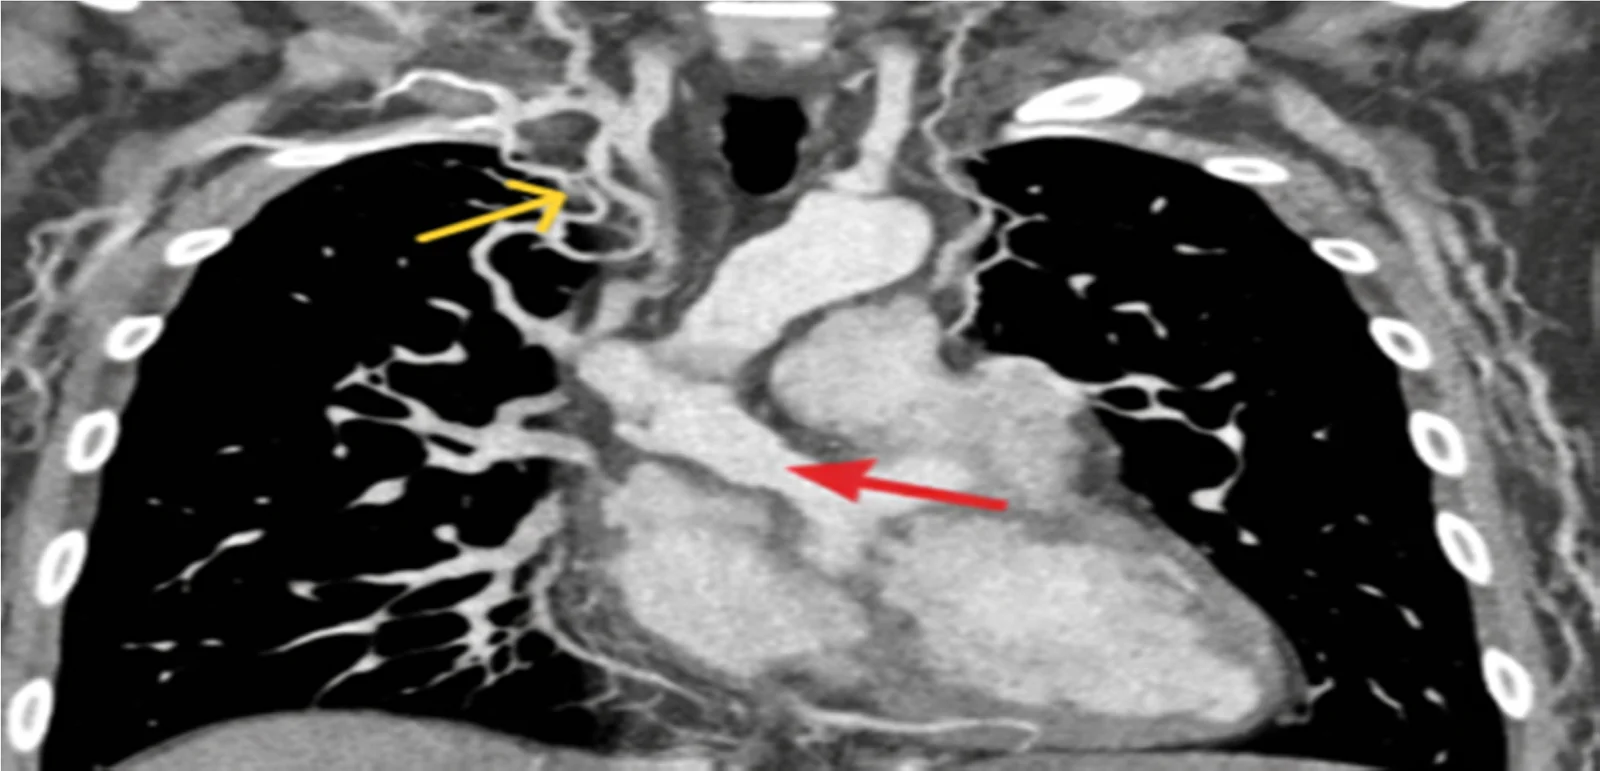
Coronal contrast-enhanced CT pulmonary angiography (CTPA) demonstrating a filling defect in the left lower lobar pulmonary artery (red arrow), consistent with pulmonary embolism. The image also shows prominent cervicothoracoabdominal collateral venous circulation (yellow arrow), reflecting chronic superior vena cava obstruction and extensive systemic venous rerouting.
